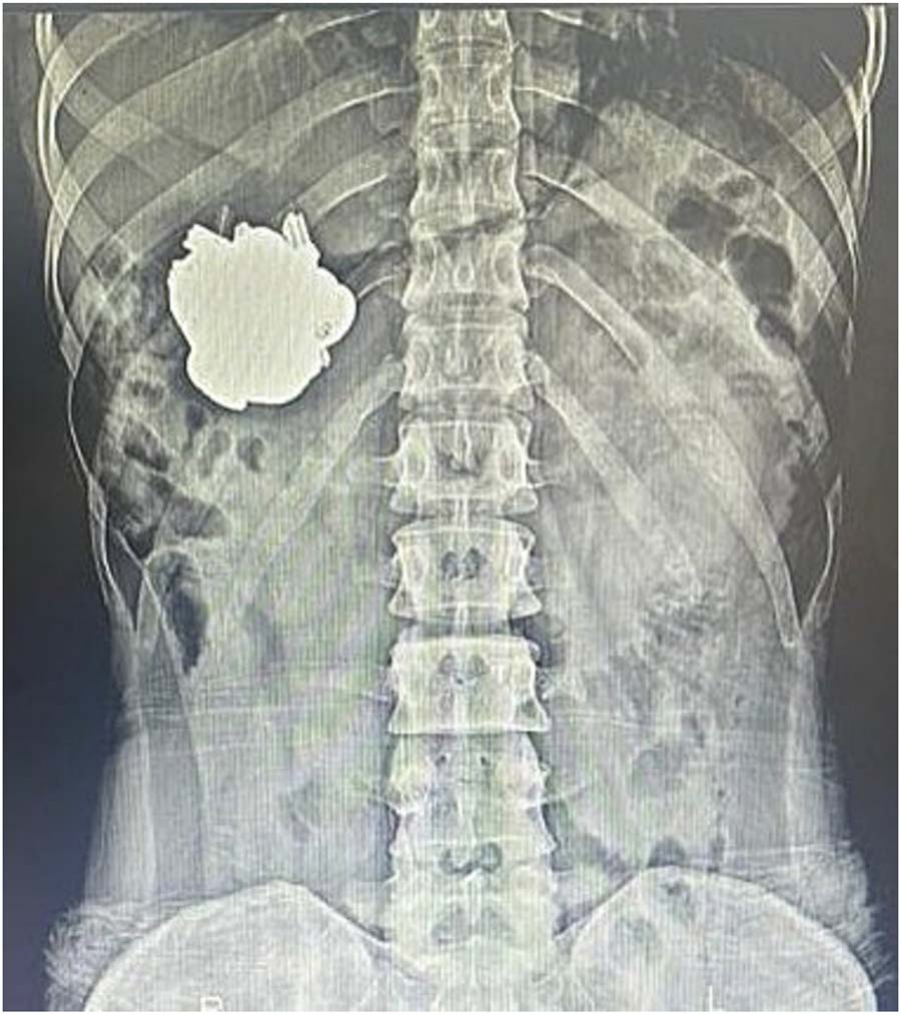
Plain abdominal X-ray (anteroposterior view) showing a large metallic foreign body projected over the left upper quadrant, corresponding to the stomach. The object has well-defined borders and dense radiopacity, consistent with an ingested metallic component.
